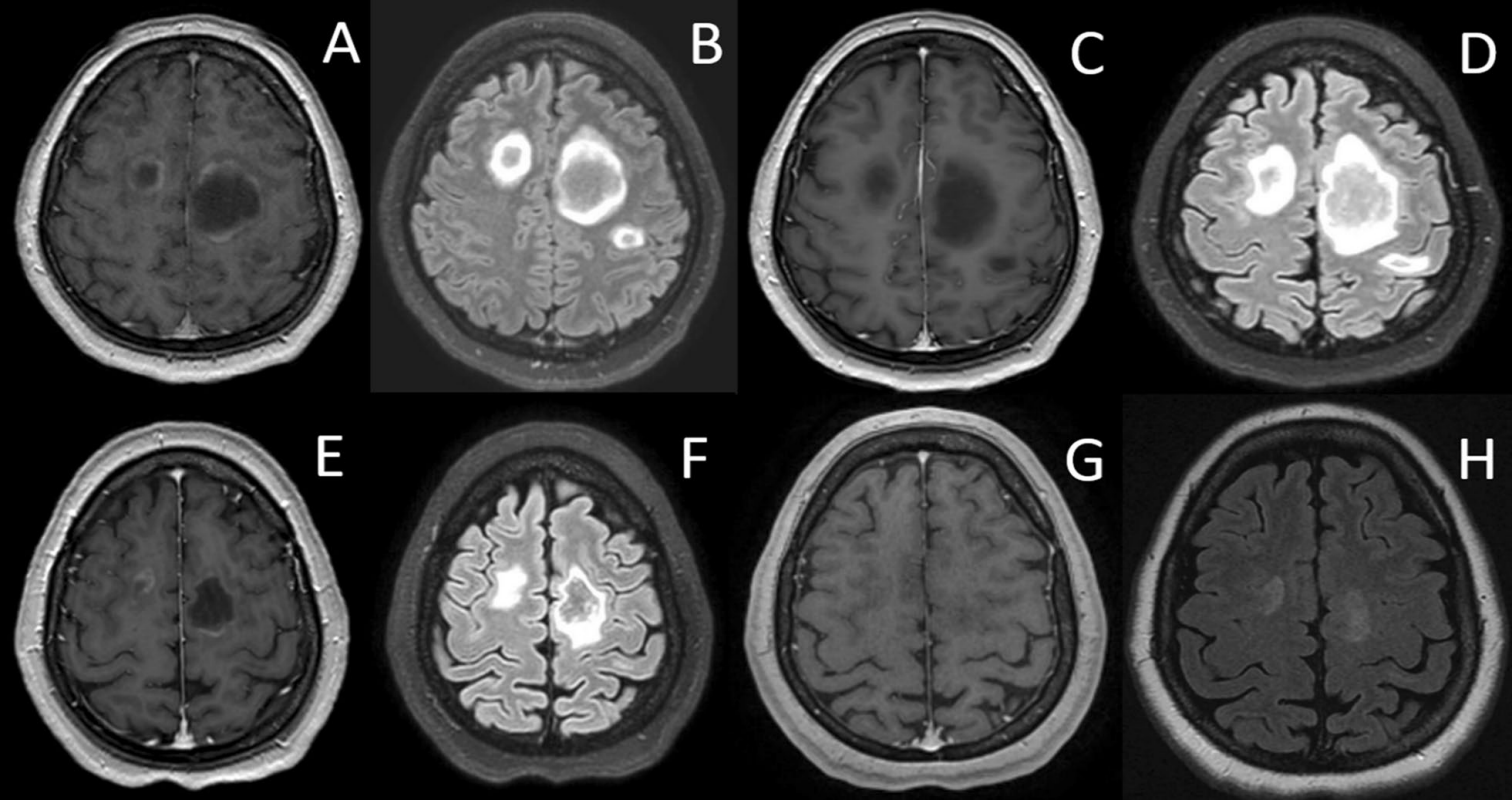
Post-contrast T1 weighted MRI (A, C, E and G) and axial T2 fluid-attenuated inversion recovery (T2 FLAIR) (B, D, F and H) during hospitalization and follow-up. First day at hospitalization (A + B), six days follow-up (C + D), five weeks follow-up (E + F) and four months follow-up (G + H)
